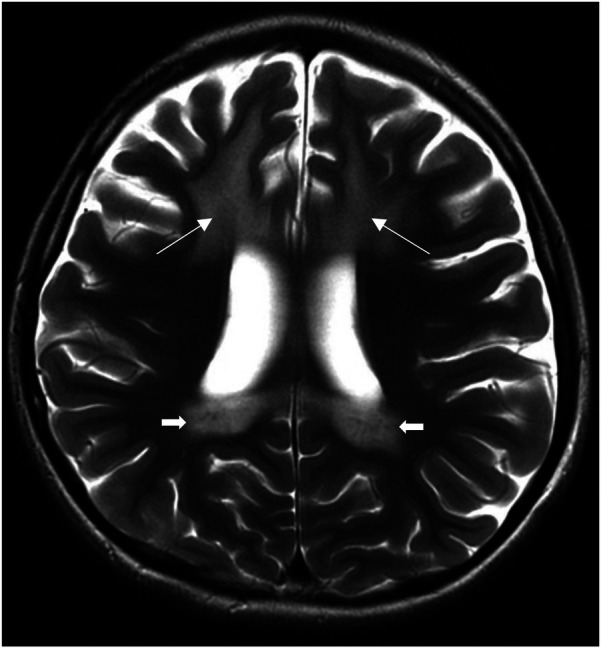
Axial T2-weighted MRI brain images at presentation showing symmetrical T2-weighted hyperintense signals of the splenium, internal capsule (arrows).
